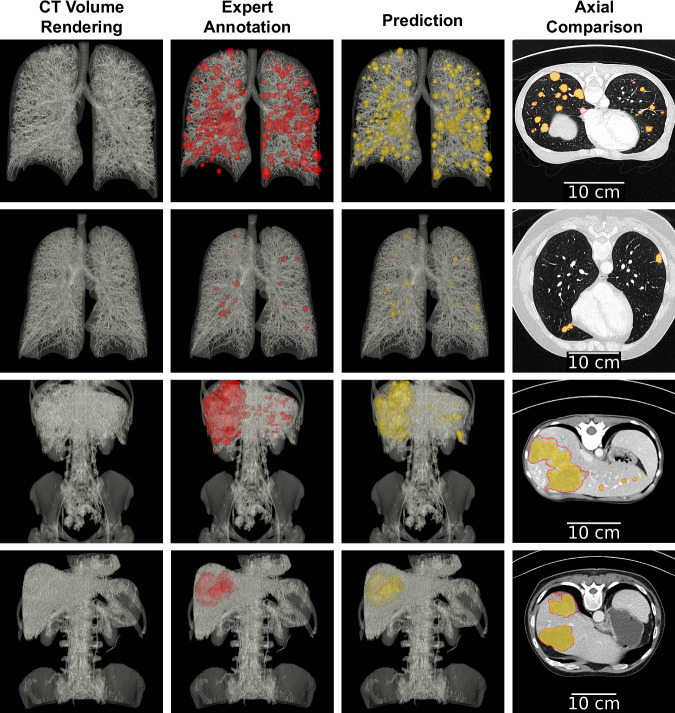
Segmentations produced by the nnUNet fine-tuned on the HCUCH dataset for automatic lung and liver tumor segmentation. From left to right, the first three columns show: (1) 3D rendering of the CT volume from the coronal view, (2) CT overlaid with expert annotations (red), and (3) CT overlaid with nnUNet predictions (yellow). The fourth column presents an axial slice with expert annotation contours and the filled predicted mask. Each row represents a different patient from the test set; the first two correspond to thoracic series and the last two to abdominal series.
